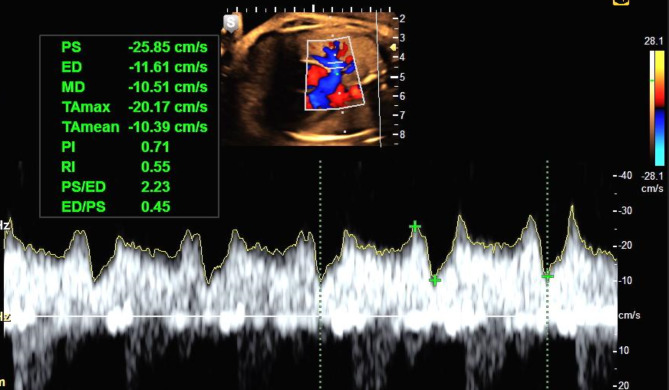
The measurement of the pulmonary vein Doppler and the Doppler waveform
